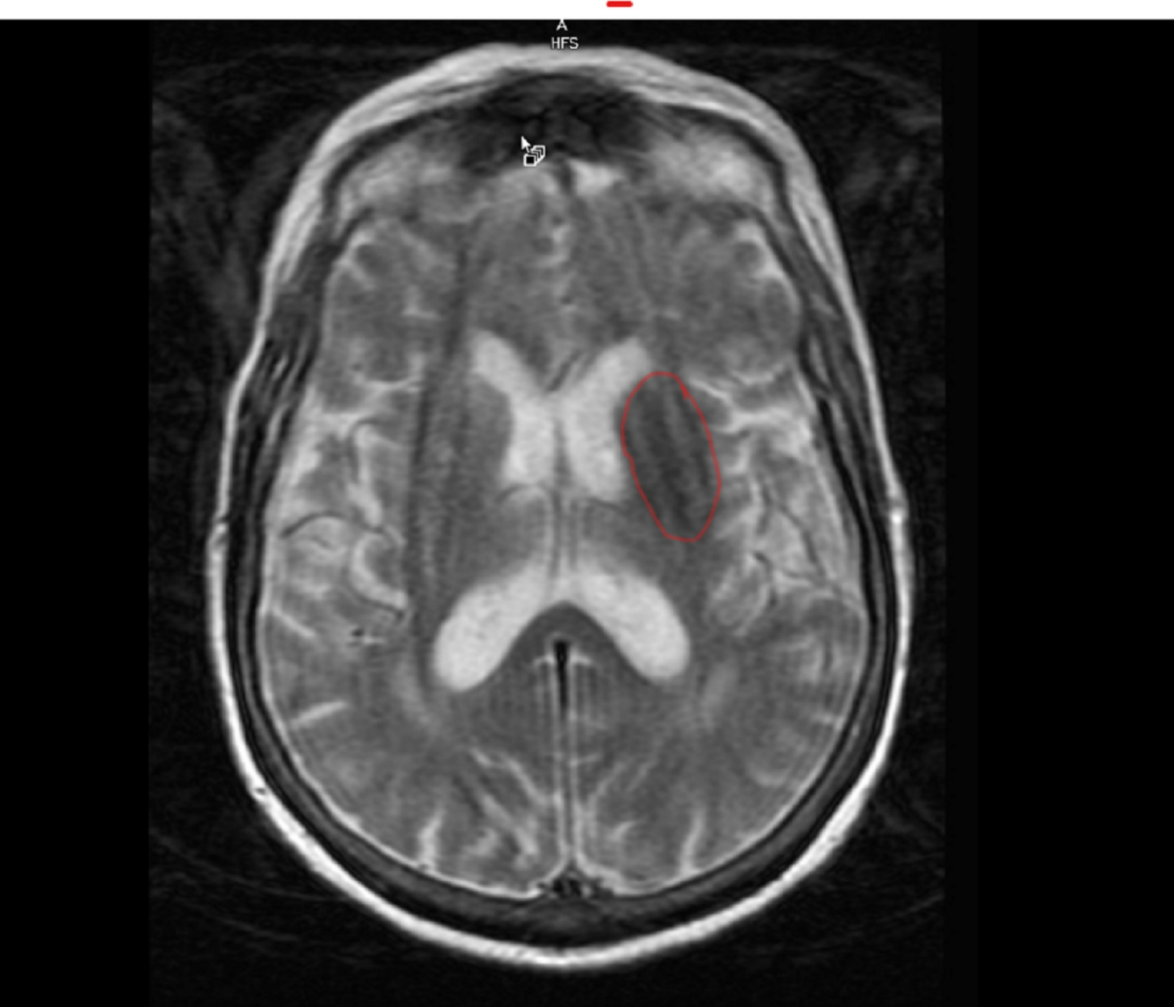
MRI brain T2 sequence showing hypodensity in left basal ganglia (area in red circle).
